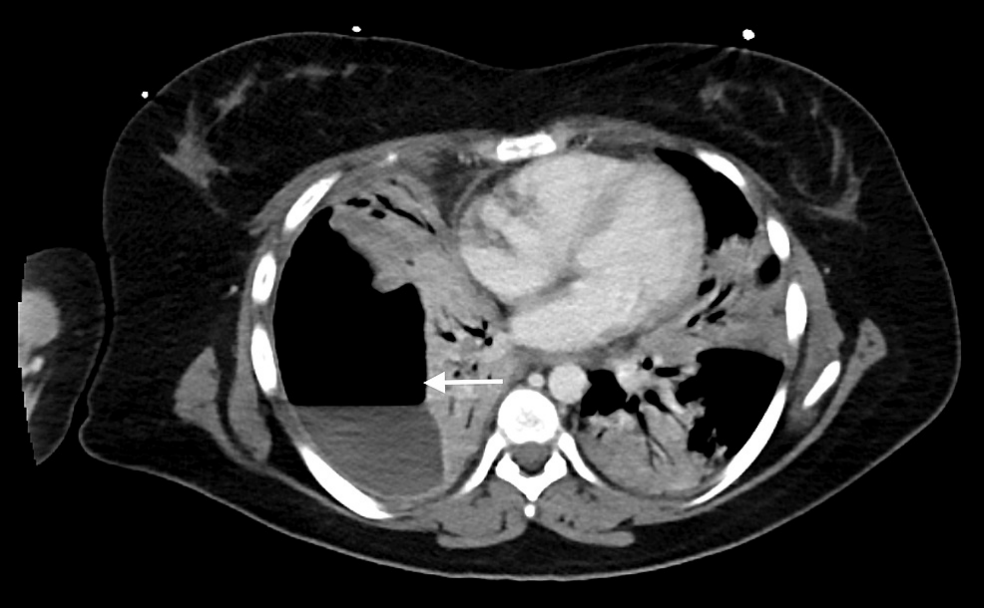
Chest CT prior to insertion of right-sided chest drain showing large hydropneumothorax (arrow)
